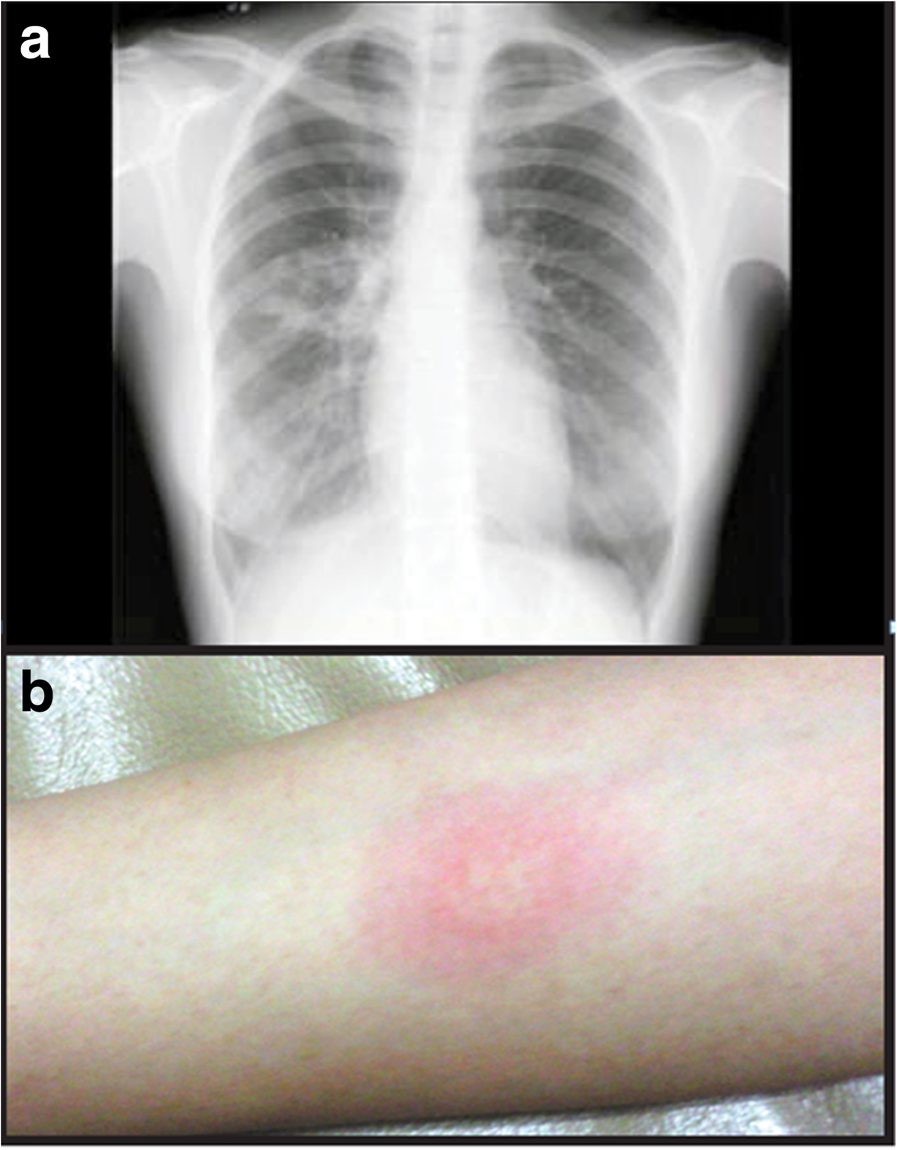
a Chest X-ray of the patient showing patchy perihilar infiltrates. b Purified protein derivate skin test showing induration of 22 mm, 72 hours after intradermal injection
